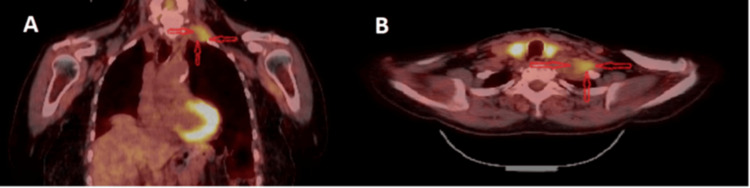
A) The PET (Positron emission tomography) coronal section shows the localization of the lesion and its corresponding FDG (Fluorodeoxyglucose) uptake, providing a clear view of the tumor’s metabolic activity and spatial relationship with surrounding structures. B) The PET axial section provides a detailed visualization of the lesion’s localization and FDG uptake, offering additional insight into the tumor’s characteristics and its interaction with nearby tissues.
